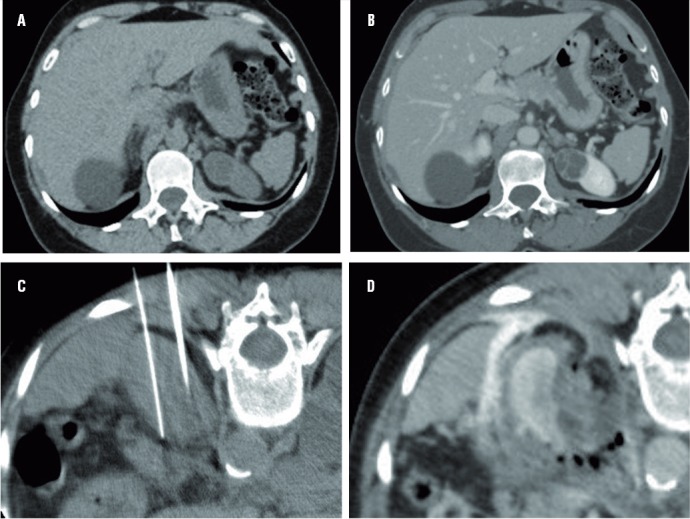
(a-d) Tomographic images of radiofrequency ablation of Bosniak IV cystic lesion. CT without intravenous contrast (a) and post contrast (b) shows the hypoattenuation lesion in the left kidney and the exophytic cortical cyst in the upper third of the left kidney, containing thick internal septa that enhances after contrast. Non-enhanced CT (c) shows the positioning of the cluster needle in the axial plane. Enhanced-CT (d) immediately after the ablation shows the volumetric reduction of the lesion and the margins obtained after contrast injection, confirming proper treatment during the procedure.
